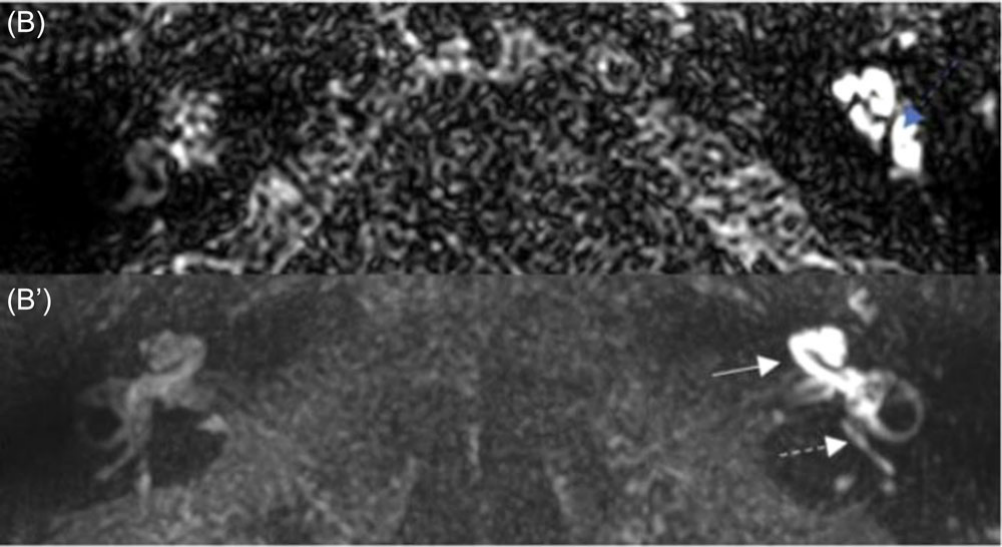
Case 3, postoperative magnetic resonance imaging. (B) No visible saccule on the left‐operated ear (blue dashed arrow). (B′) Intense blood‐labyrinth barrier impairment of the cochlea (white arrow), the vestibule, and semicircular canals (white dashed arrow).
